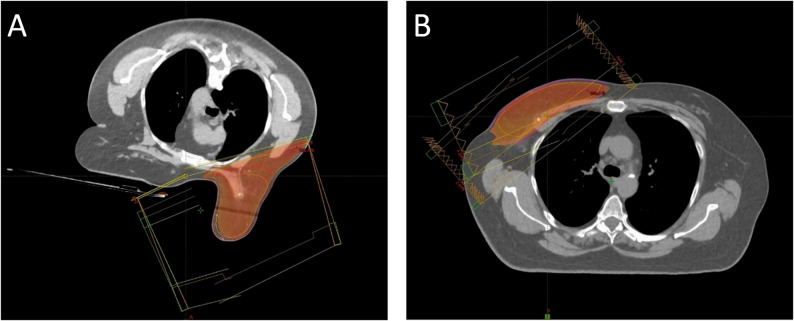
CT-scan of a patient from the investigated cohort. (A) Prone position; (B) Supine position. Note: The orange line indicates clinical target volumes (CTV), and the red line indicates planning target volumes (PTV). The orange area represents the applied dose of the radiation plan. White lines indicate irradiation-fields
